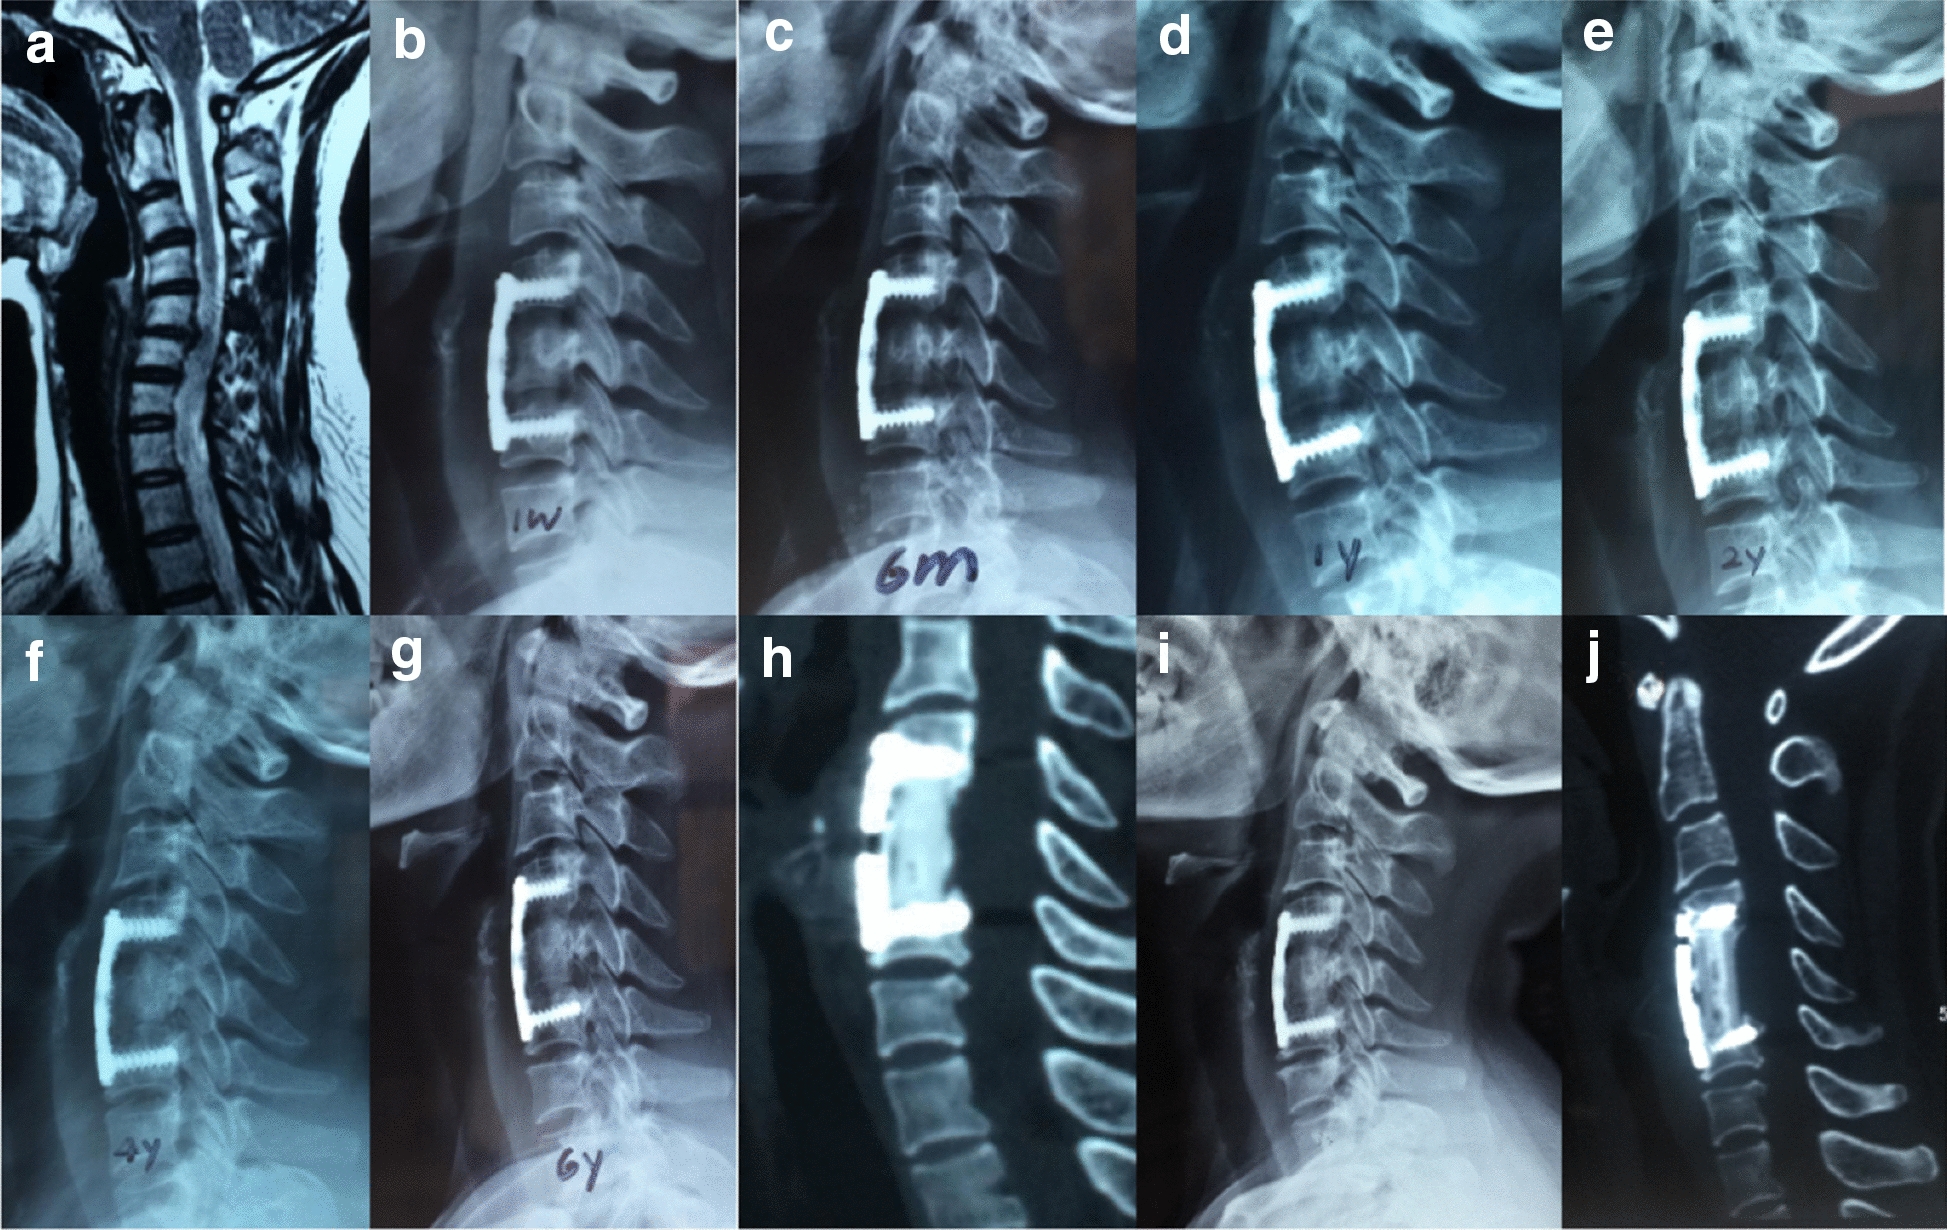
Type a bone fusion. a–k A 43-year-old woman whose preoperative cervical MRI (a) revealed C5/6 disc herniation underwent 1-level corpectomy with an n-HA/PA66 strut (b). Lateral X-ray films indicated bony fusion of the autogenous bone granules filling the strut at the 3-month, 6-month, 12-month, 2-year, 4-year, 6-year, and 8-year follow-up visits (c–g, i). 3-D CT at the 6-year and 8-year follow-up visits (h, j) showed solid bone fusion
